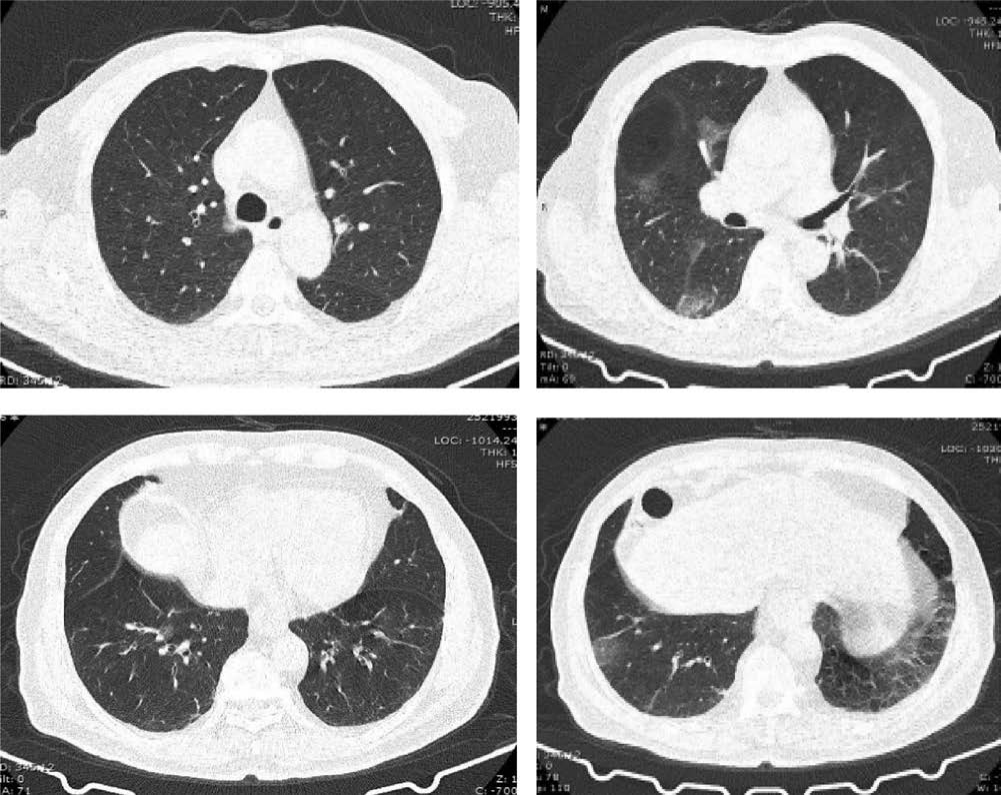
The patient tested positive for COVID-19 antigen, and chest CT showed multiple ground-glass opacities in the right lung.
